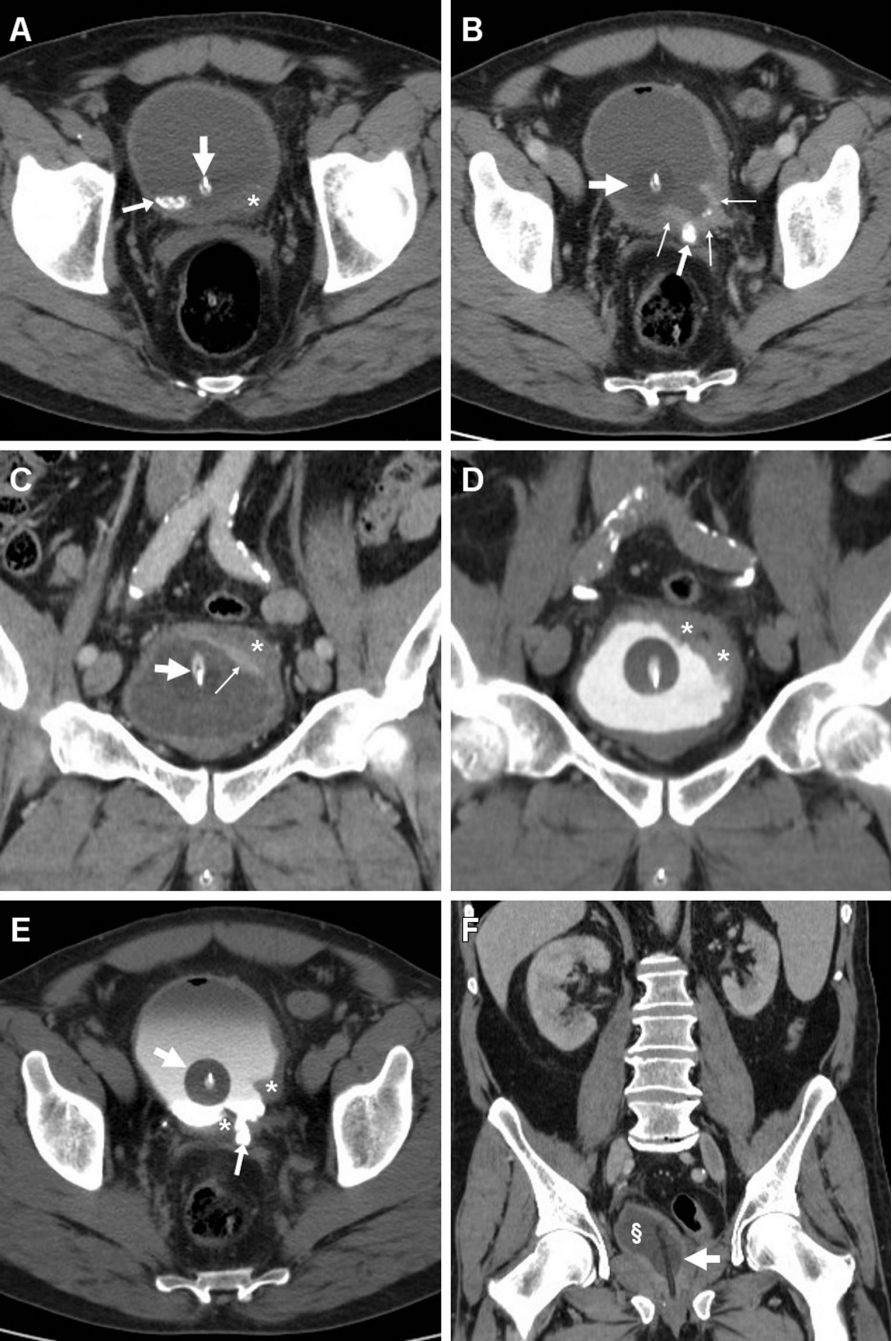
Muscle-invasive bladder carcinoma in a 54-year-old man with urolithiasis (*arrows*) and long-term bladder catheterisation (*thick arrows*). Unenhanced (a), portal (b, c) and excretory (d, e) phase CT images showed focal solid mural thickening (*) at the left posterolateral bladder wall, with an irregular configuration and positive contrast enhancement (*thin arrows*). Postoperative status after radical cystectomy (f) with orthotopic neobladder (§) is shown on follow-up CT (f)
